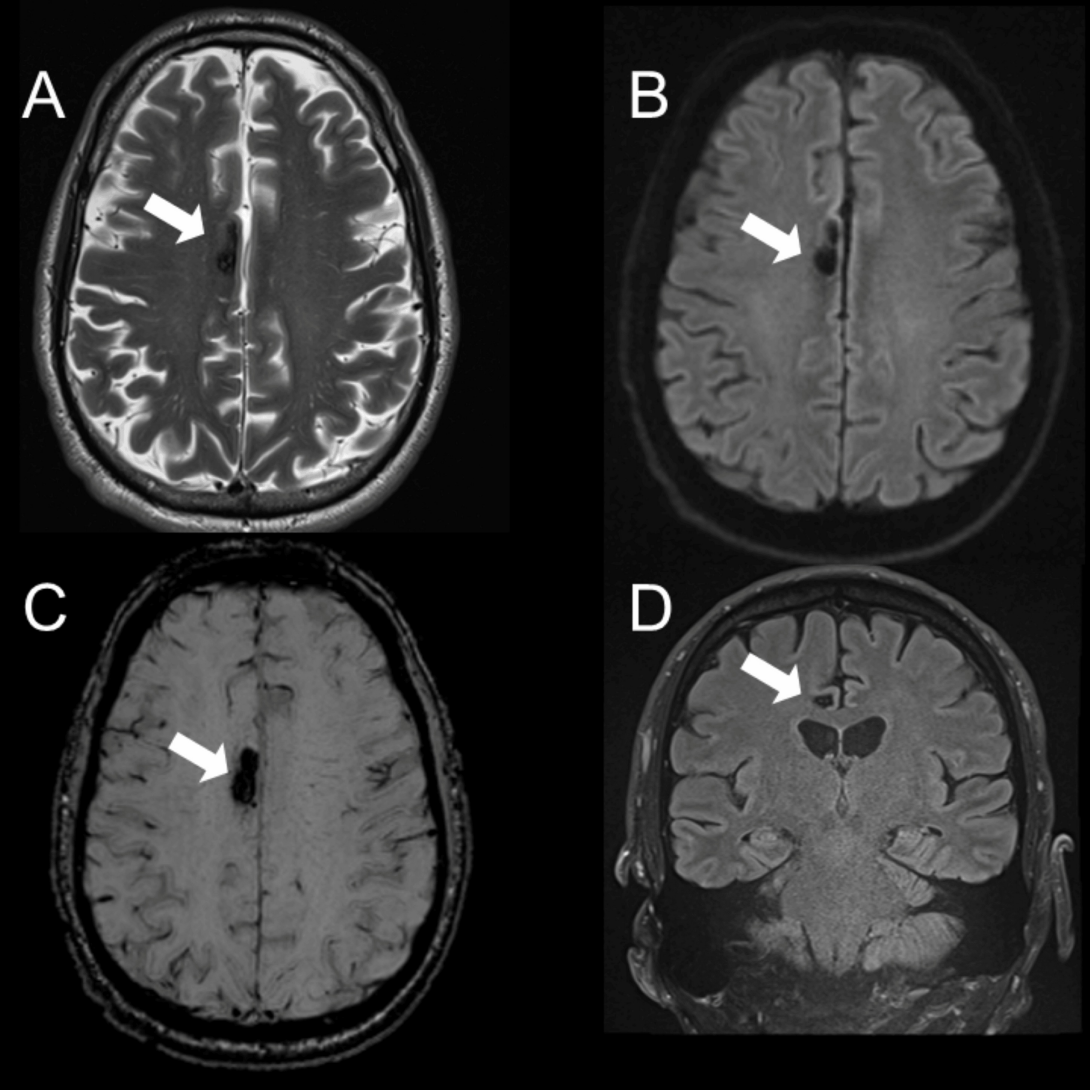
Brain MRI images T2-weighted (A), FLAIR (B, D), and SWI (C) reveal a right frontal cerebral cavernous malformation located just above the corpus callosum (marked by arrow). The lesion exhibits high signal intensity on T2 and FLAIR (A and B) with a surrounding rim of low signal intensity. Additionally, SWI (C) displays a blooming artifact characteristic of cavernoma. FLAIR: fluid-attenuated inversion recovery; SWI: susceptibility-weighted imaging
